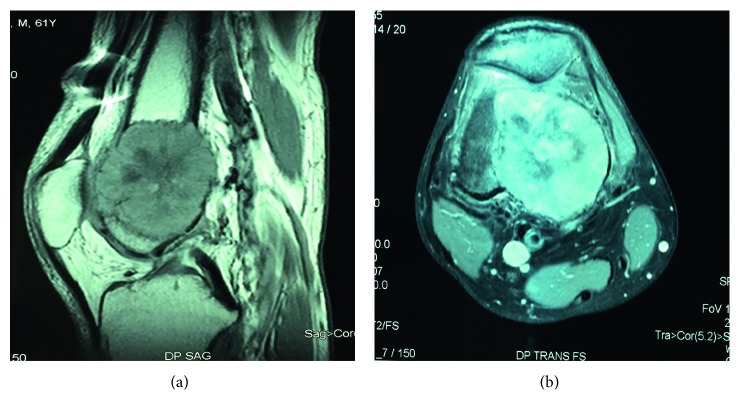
Sagittal and transversal MRI sections of the right knee showing plasmacytoma of the lower right femur metaphysis blowing the inner cortical.
